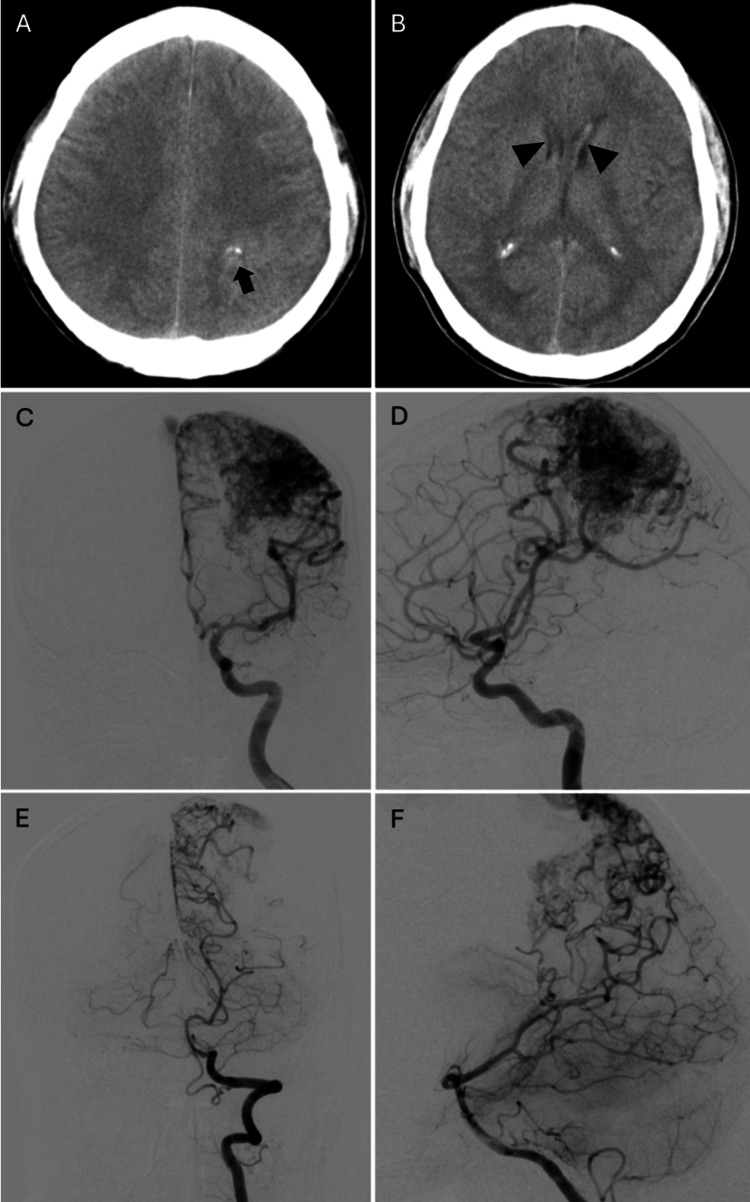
Initial cranial CT scan and cerebral angiography CT: computed tomography; AP: anteroposterior; AVM: arteriovenous malformation (A-B) Sequential axial CT images demonstrate a superficially located, ill-defined, mildly hyperdense lesion in the left parietal lobe with internal calcifications (arrow) and associated intraventricular hemorrhage (arrowheads) involving both lateral ventricles. (C) AP and (D) lateral views of a left internal carotid artery injection and (E) AP and (F) lateral views of a left vertebral artery injection reveal a large AVM located in the left parietal region
